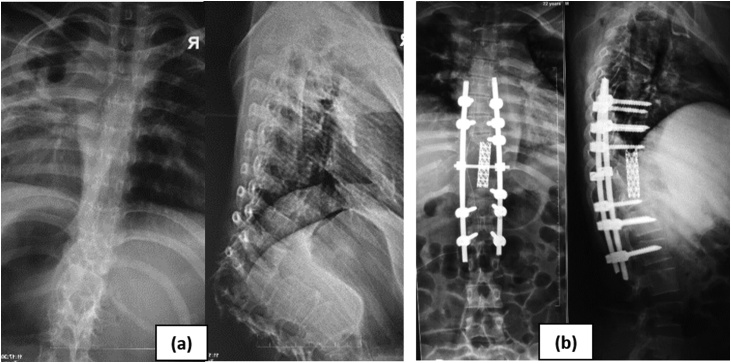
Kyphotic correction with modified lateral extracavitary approach. (a) Preoperative Xray-MRI with local/regional kyphotic 90.30, and lesion at Th11-L1 vertebrae due to spondylitis tuberculosis. (b) Post operative Xray with correction local/regional kyphotic 26.50.
